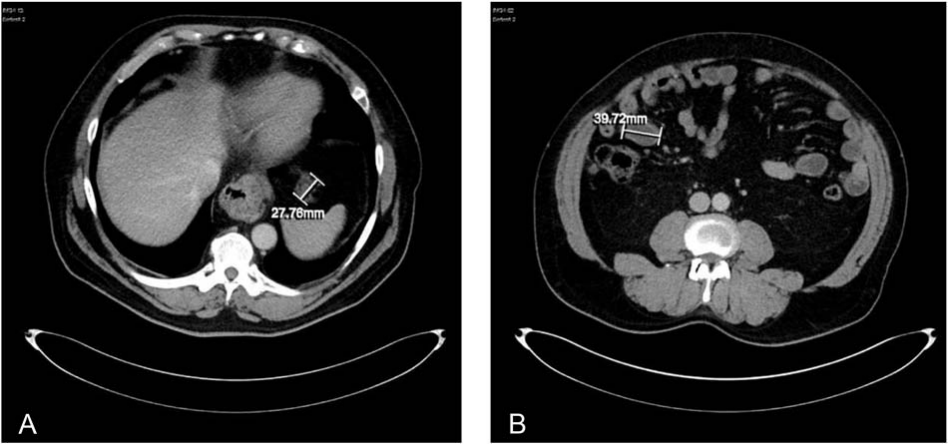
(A) axial CT abdomen demonstrating a serosal deposit measuring 27.76 mm. (B) Axial CT abdomen demonstrating a peritoneal abdominal wall deposit measuring 39.72 mm.
